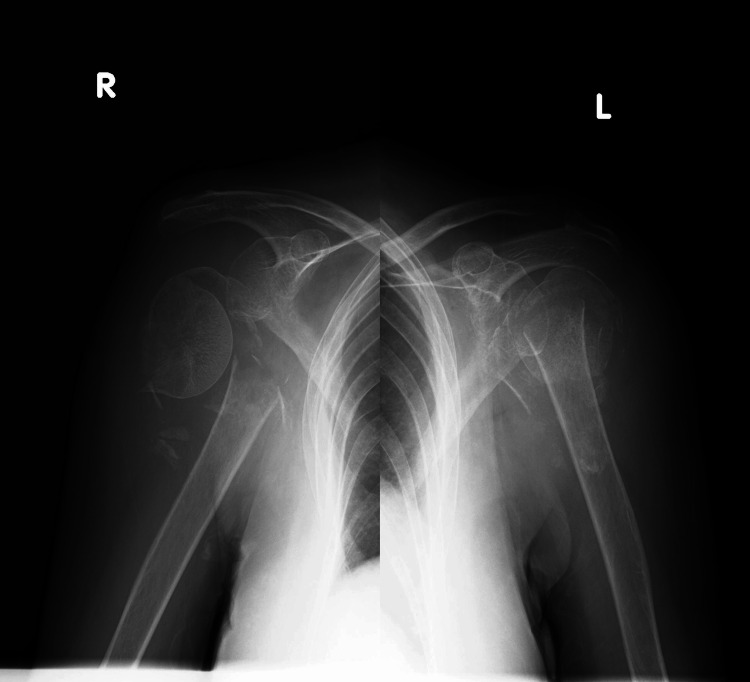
Preoperative radiograph of both shoulders Preoperative anteroposterior X-ray images of both shoulders.
